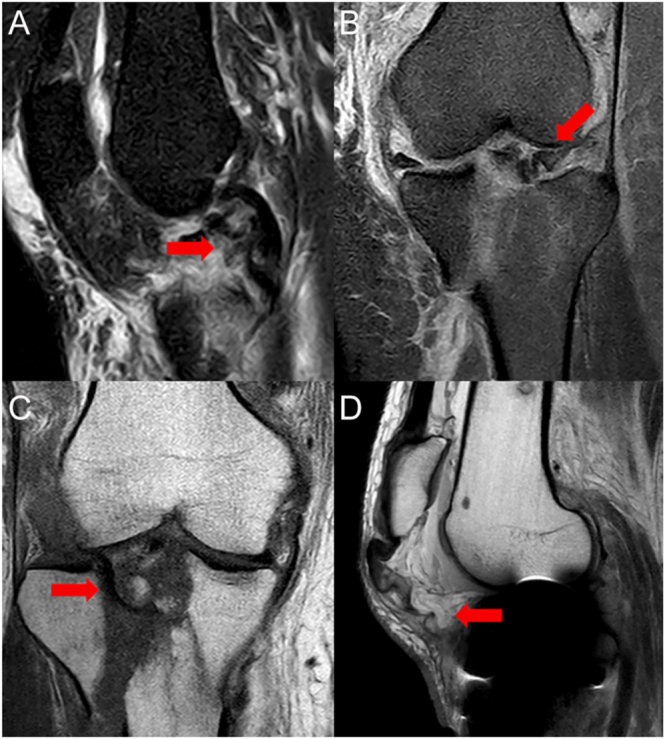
MRI may be useful to identify intra- and extra-articular pathology that may require concomitant surgery. (A) Bucket-handle tear of the lateral meniscus displaced into the intercondylar notch (sagittal plane). (B) Bucket-handle tear of the lateral meniscus displaced into the intercondylar notch (coronal plane). (C) Lateral meniscus interposed within the fracture site, preventing proper reduction of the fracture. (D) Confirmation of patellar tendon detachment from the anterior tibial tuberosity.
